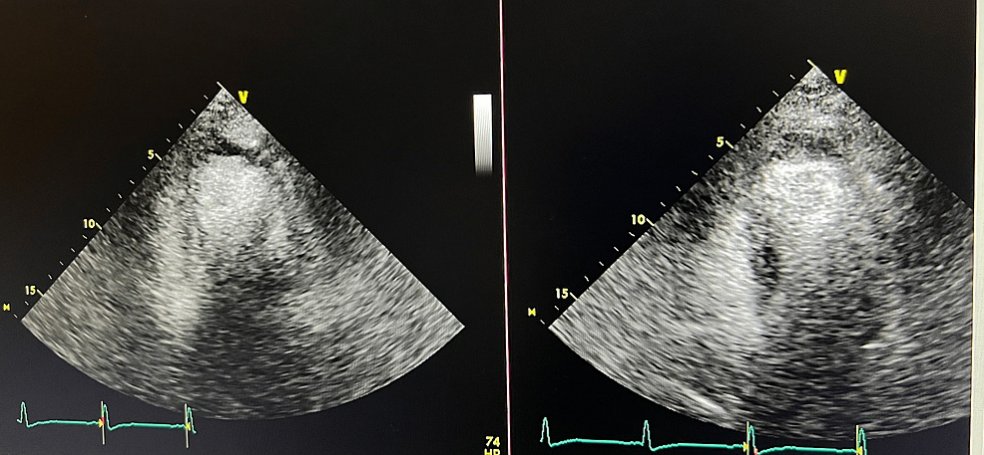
Transthoracic echocardiography four-chamber view showing reduced EF at the end of systole and diastole, respectively EF; ejection fraction
